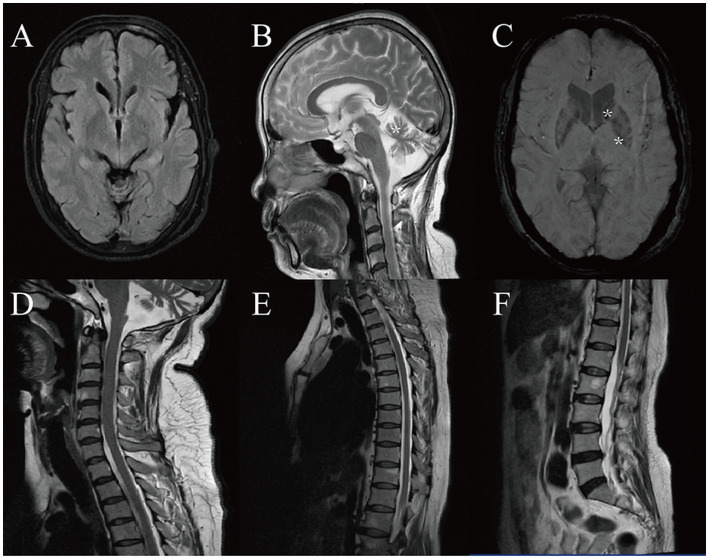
Brain and spine magnetic resonance imaging (MRI) of the patient. (A) Fluid attenuated inversion recovery image, showing mild global cerebral atrophy. (B) Sagittal view of T2-weighted image, showing marked cerebellar atrophy (*). (C) Susceptibility-weighted image, showing iron deposition in the caudate nucleus and globus pallidus (*). (D) T2-weighted cervical spine MRI. (E) T2-weighted thoracic spine MRI. (F) T2-weighted lumbar spine MRI.
